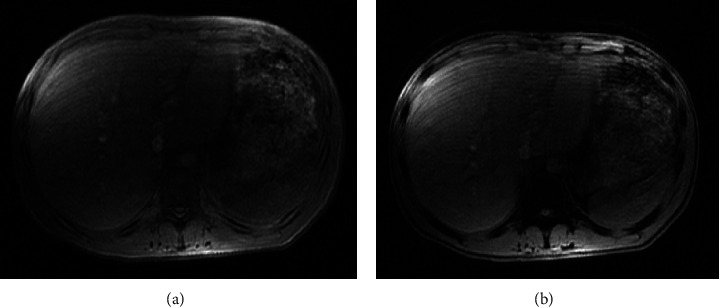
T1-MRI images showing no difference in the signal intensities between the in-phase (a) and opposed-phase (b).
